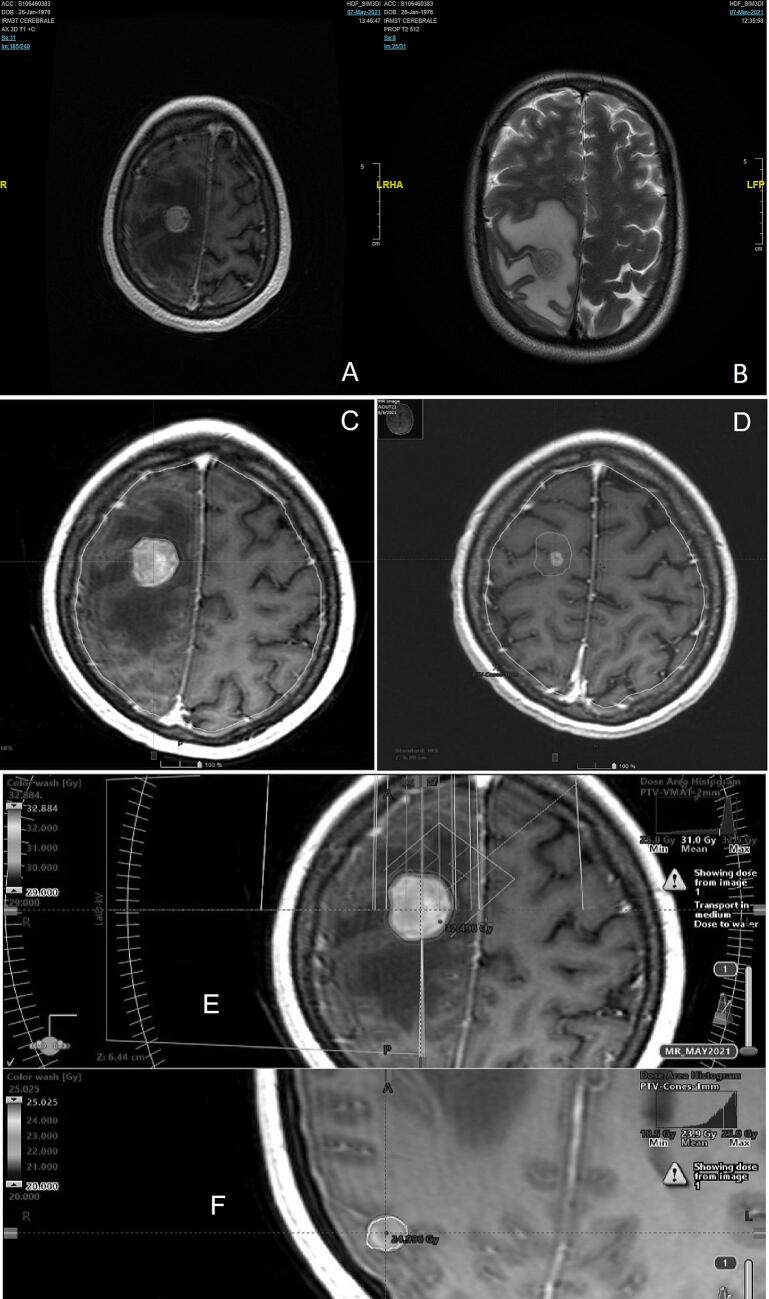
Brain MRI with gadolinium showing right parietal and fronto-parietal lesions with surrounding vasogenic edema (A&B). Treatment dosimetry in color wash representation at the right parietal metastasis (E) and the right fronto-parietal metastasis (F). Radiological response after SRT: Pre-SRT axial T1-weighted gadolinium-enhanced brain MRI showing the 20x18x21 mm right fronto-parietal (C), 10 weeks post-SRT axial T1-weighted gadolinium-enhanced brain MRI showing the extent of response at the level of the same lesion with complete resolution of the surrounding vasogenic edema (D).
